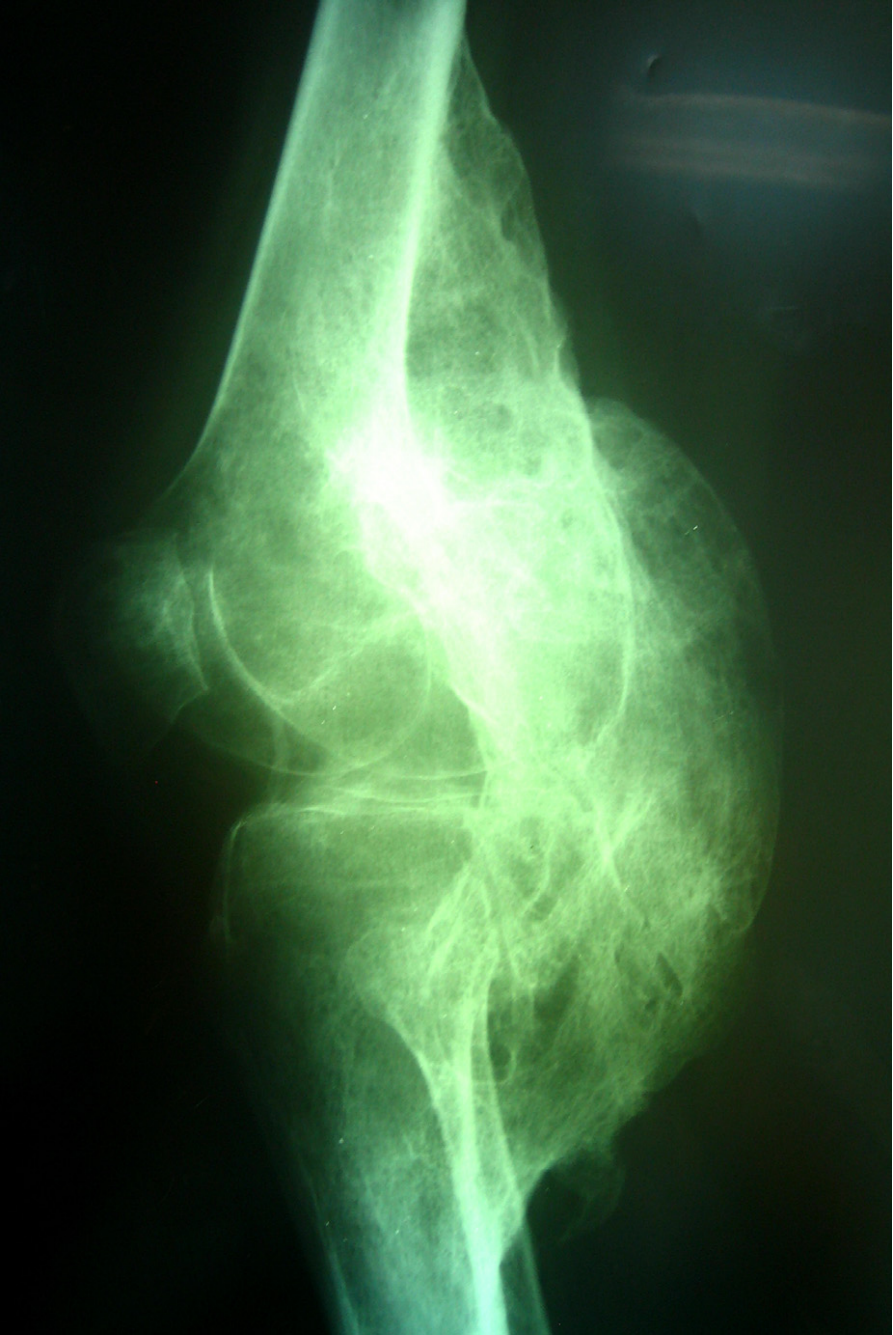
Lateral radiography of the right knee, large mass of heterotopic bone is seen bridging the knee joint posteriorly.
